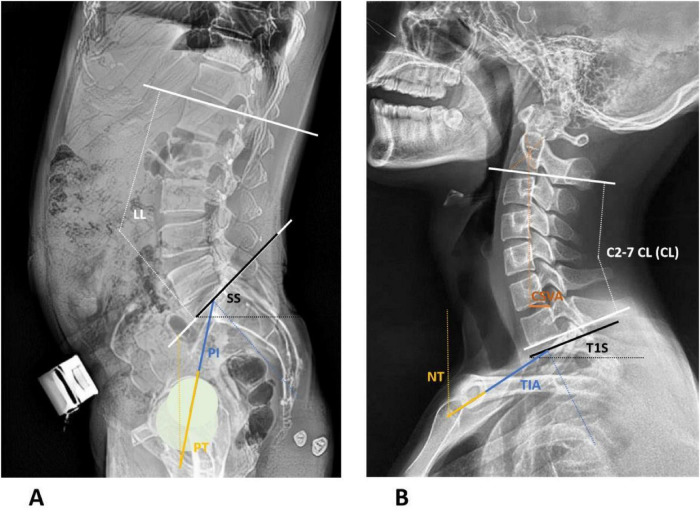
Radiographic measurements of sagittal spinal parameters. (A) Lumbar spine and pelvis: LL (lumbar lordosis), SS (sacral slope), PI (pelvic incidence), PT (pelvic tilt). (B) Cervical and upper thoracic spine: C2-7 CL (cervical lordosis, defined as the angle between the superior endplate of C2 and the inferior endplate of C7), cSVA (cervical sagittal vertical axis, defined as the horizontal distance between the posterior superior corner of C2 and a vertical plumb line dropped from the posterior superior corner of C7), T1S (T1 slope, defined as the angle between the superior endplate of the T1 vertebra and a horizontal reference line), TIA (thoracic inlet angle, defined as the angle between the line parallel to the T1 superior endplate and the line connecting the T1 superior endplate to the center of the sternoclavicular joint), NT (neck tilt, defined as the angle between the vertical line and the line connecting the T1 superior endplate to the center of the sternoclavicular joint).
